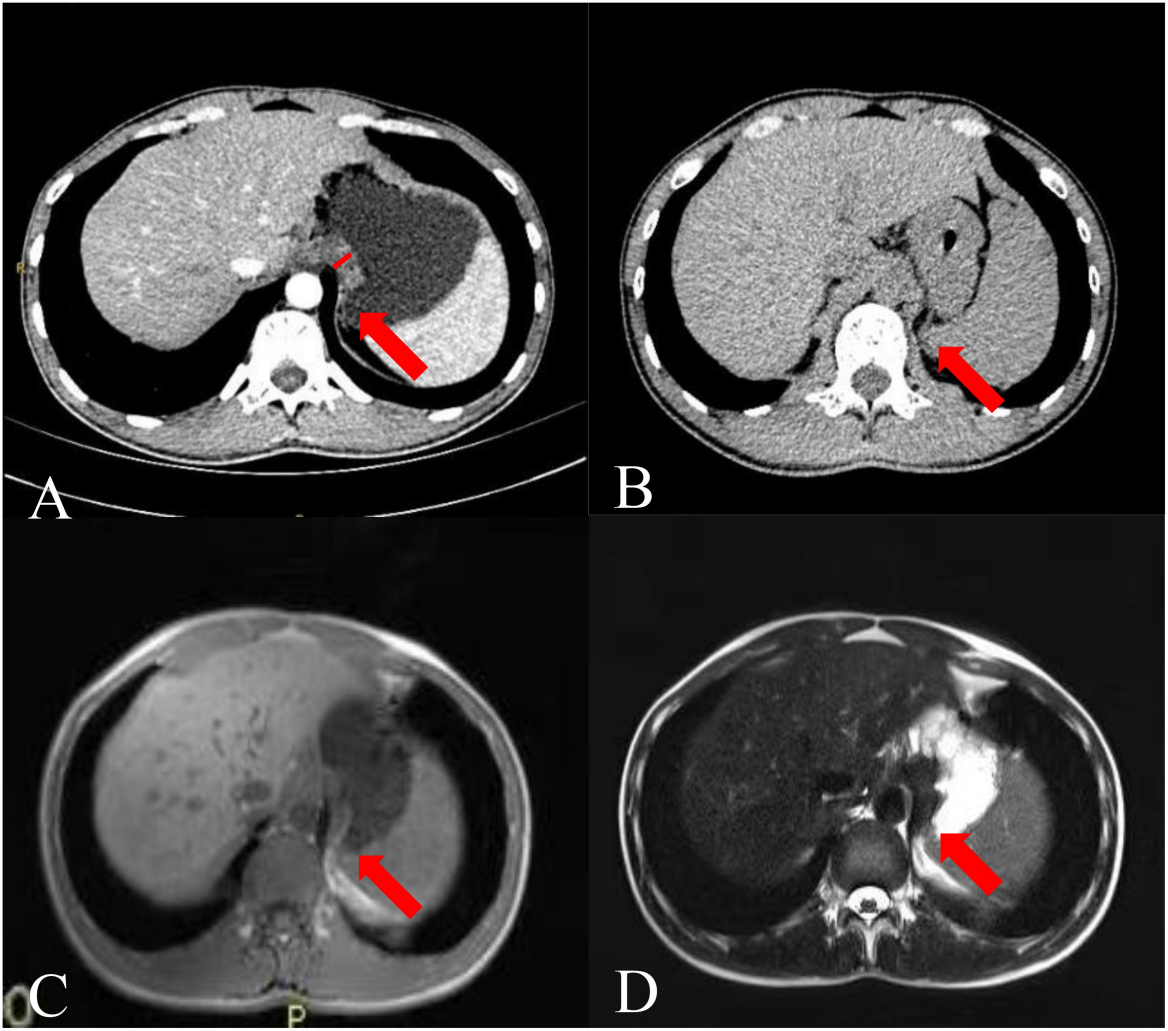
Images of a 26-year-old man. (A) CTE image showing thickening of the gastric fundus. The arrow points to the TDC, and the white vertical line indicates the TDC. (B) Single-phase upper abdominal CT image showing that the TDC was similar to that of the gastric fundus on CTE. (C) Single-phase gastric MR T1 weighted image and (D) T2 weighted image showing that the thickened part of the gastric fundus was the impression of the diaphragm crura.
